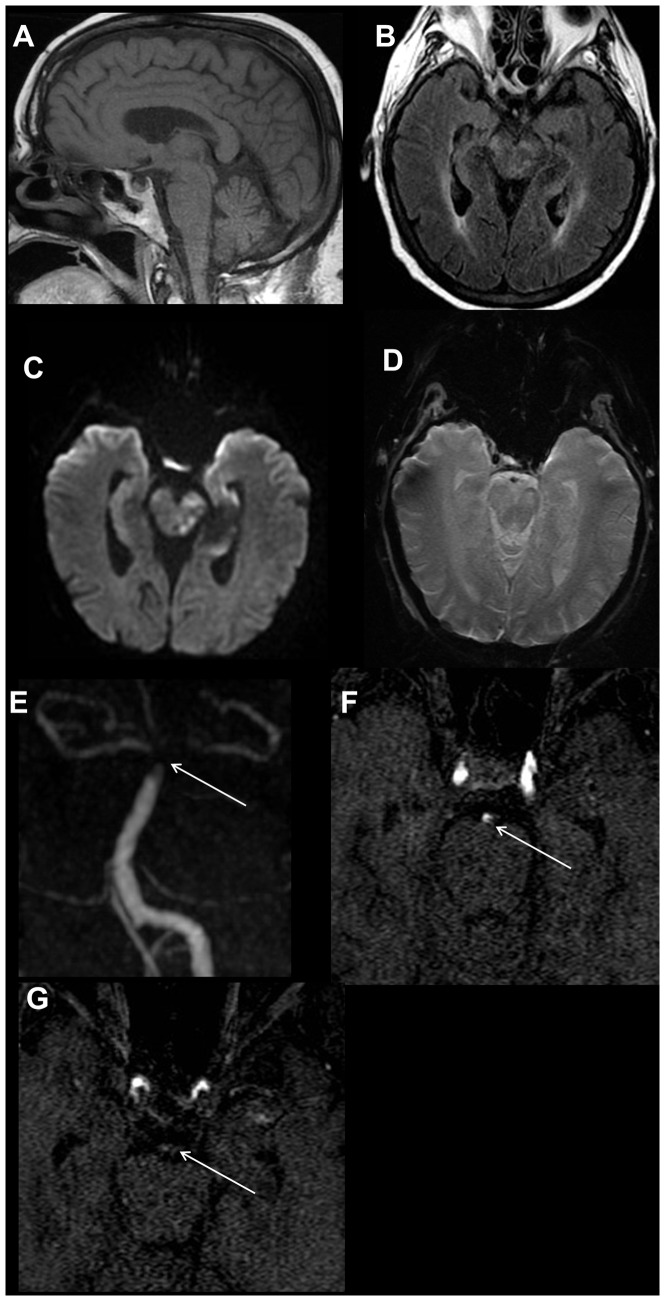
72-year-old female with altered mental status and ischemic stroke. On the 4-sequence MRI protocol (A-D), the axial FLAIR image (B) demonstrates multifocal ill-defined areas of signal hyperintensity within the midbrain with corresponding restricted diffusion on the axial diffusion weighted trace image (C), consistent with infarctions. Additional foci of infarction were seen in the left occipital lobe, left mesial temporal lobe, both thalami and the cerebellum (images not shown). No definite abnormality is seen on the sagittal T1-weighted image (A) or axial T2* image (D). MR angiography was also requested. MRA maximum intensity projection image (E) reveals thrombus with the distal basilar (white arrow) and proximal posterior cerebral arteries, which is confirmed on the axial source MR angiography images (F, G) (white arrow). Even in retrospect, this finding was not apparent on any of the sequences included in the 4-sequence MRI protocol. MRA imaging adds significant value in cases with clinical suspicion for vascular occlusion or thrombosis.
